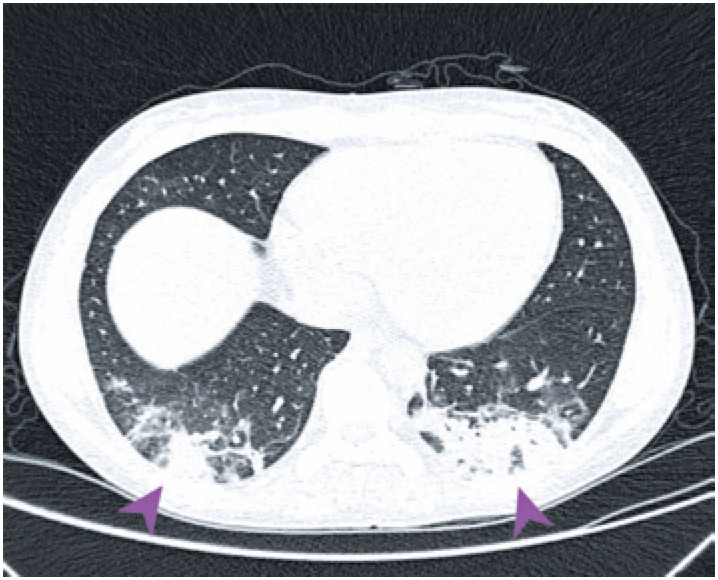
Axial chest computed tomography without contrast showing bilateral ground-glass opacities in lung bases, with peripheral and posterior predominance, suspected of being affected by COVID-19
